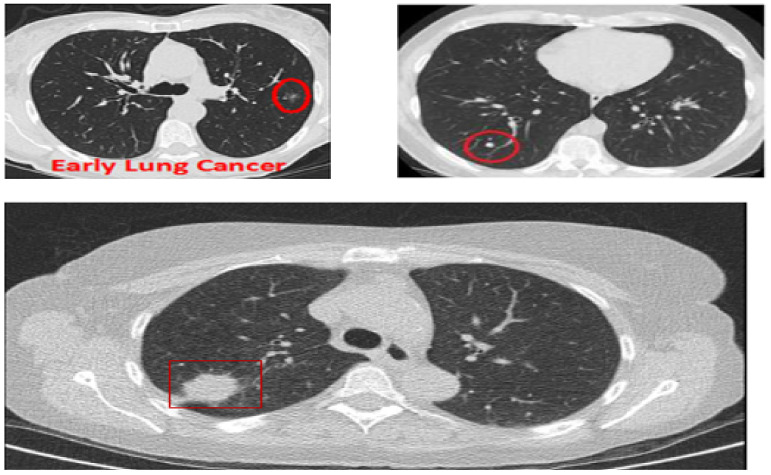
CT scans based on maximum intensity projection.
